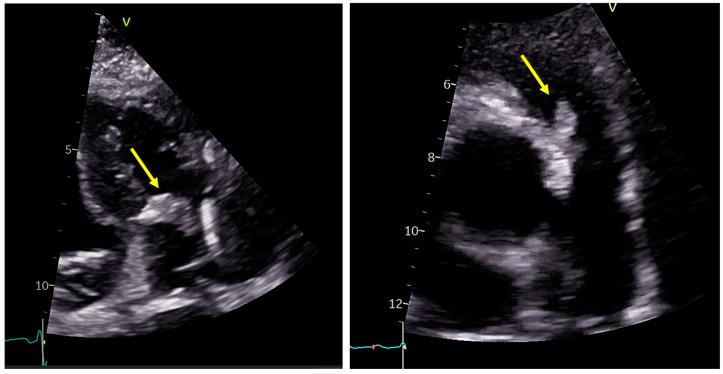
Transthoracic echocardiographic images of vegetations on the tricuspid valve (on the left) and pulmonary conduit (on the right). Yellow arrows point to vegetations.
